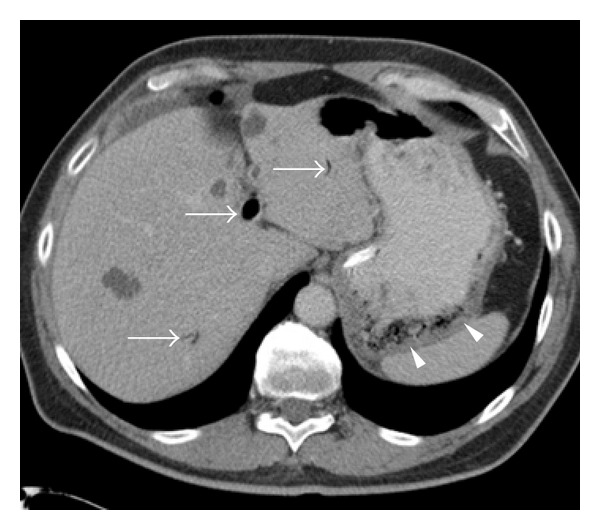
Axial CT scan image demonstrating portal venous gas (arrows) and gastric emphysema (arrowheads). Several benign hepatic cysts are also visualized.
